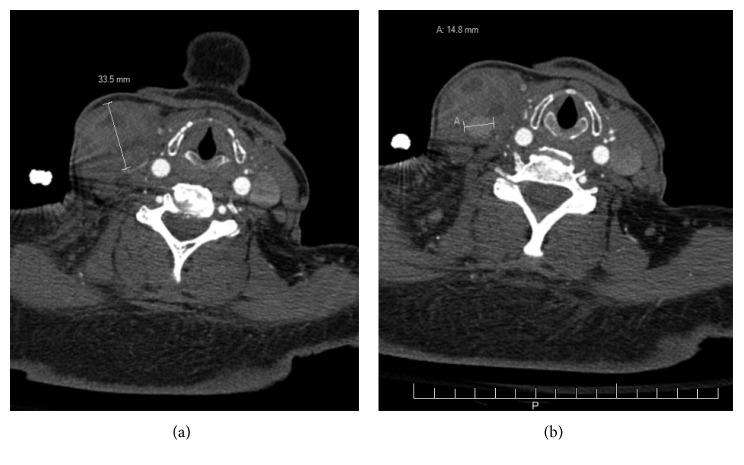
CT angiography of neck: (a) right neck mass measured and (b) right neck pseudoaneurysm measured.
